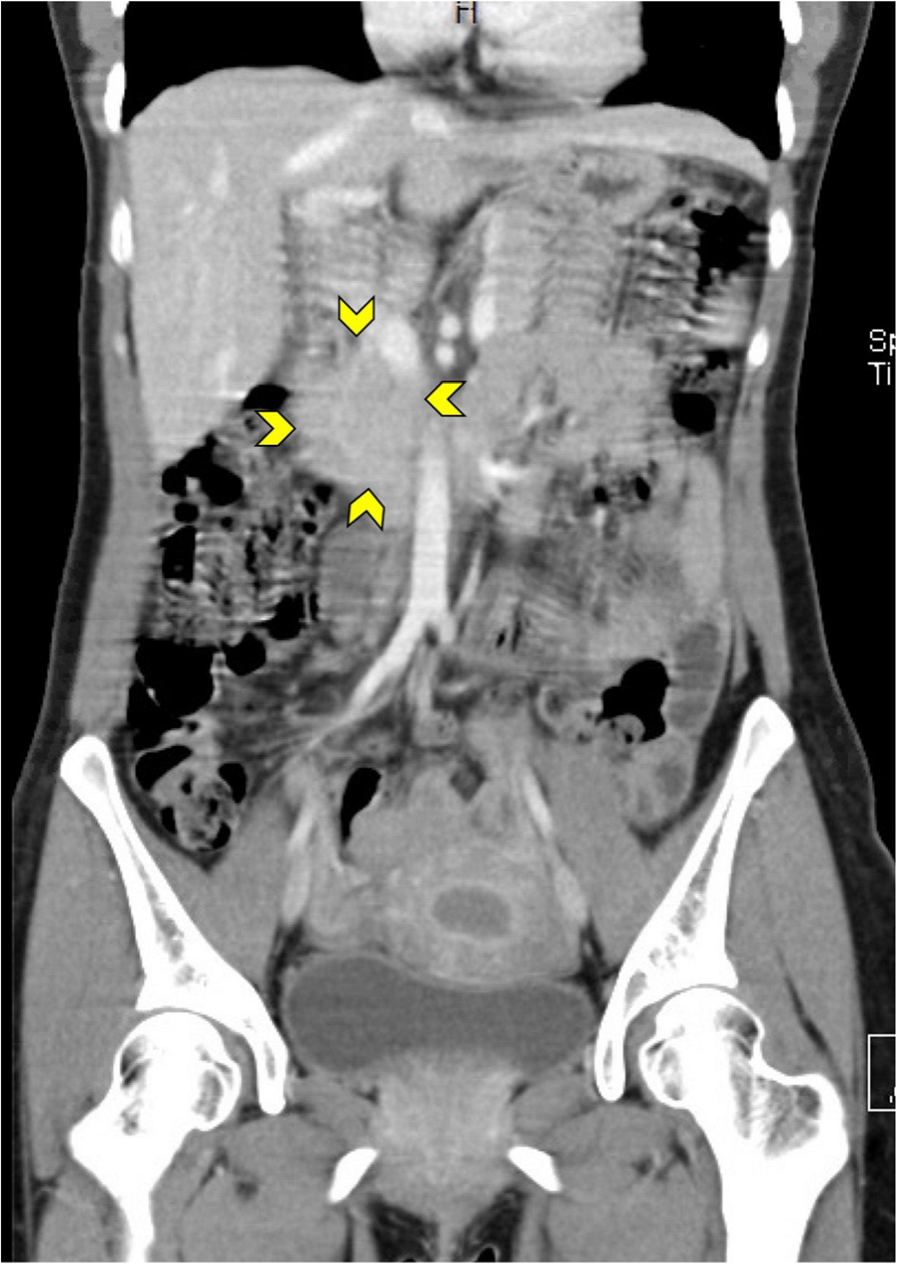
A 29-year-old woman presented with epigastric and lower abdominal pain, fresh vaginal bleeding, and weight loss. Coronal CT scan of the abdomen with intravenous contrast revealed a non-enhanced complex mass lesion measures 4 × 2.5 cm anterior and to the right of the IVC at the level of L3-L4 vertebrae suggesting matted lymph nodes (arrows). Acid Fast Bacilli smear from the mass was positive
